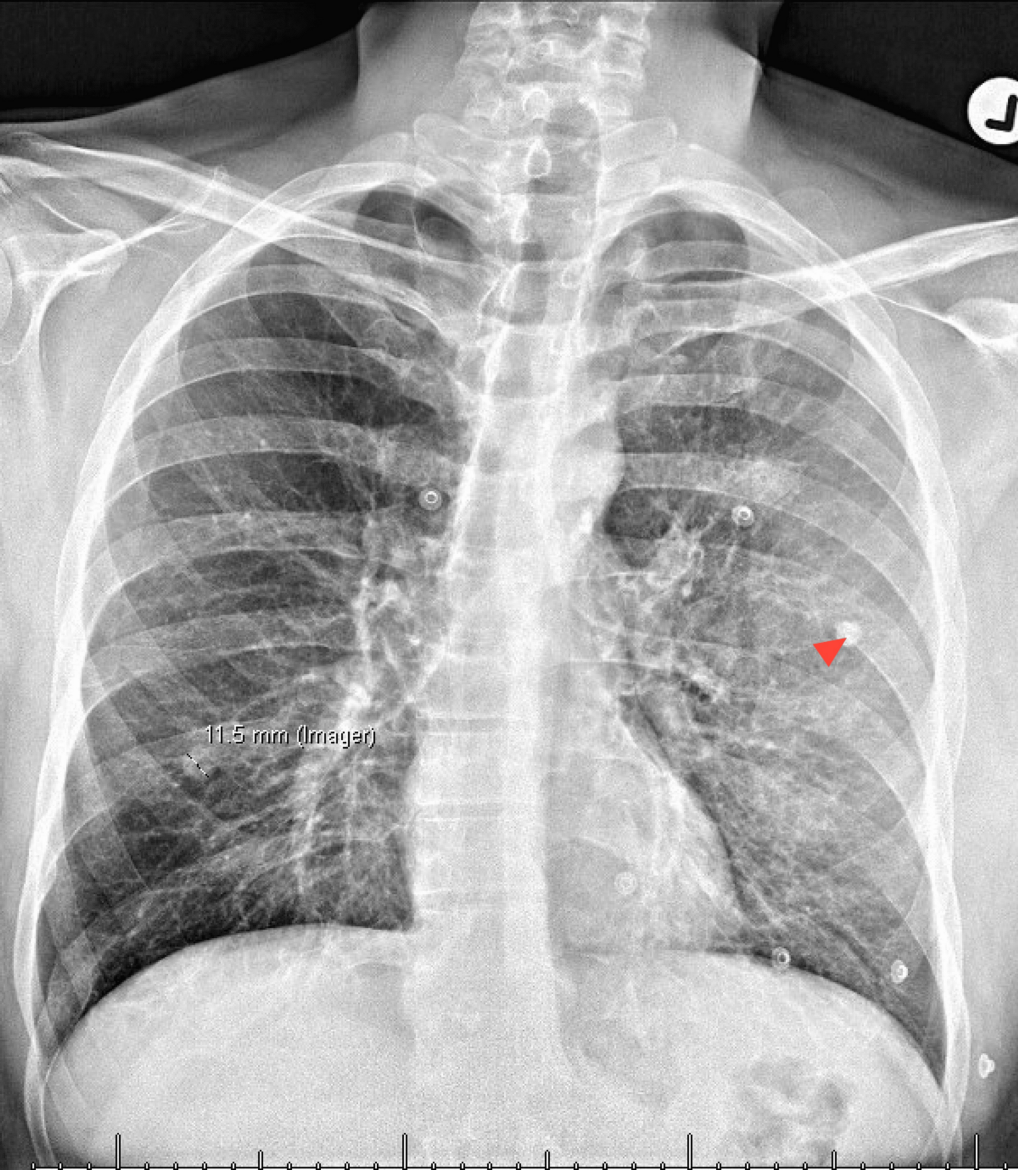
CXR PA view. The red arrowhead shows the calcified granuloma in the left lobe. CXR, chest X-ray; PA, posteroanterior.
